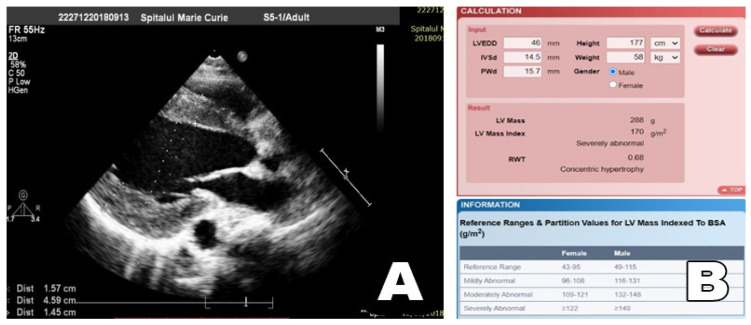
(A) Measurement of the LV wall thickness by 2D echocardiography, parasternal long-axis view (14-year-old patient from the study sample); (B)—calculation of the LVMI for the same patient (LVMI = 170 g/m2—severe concentric LVH).
